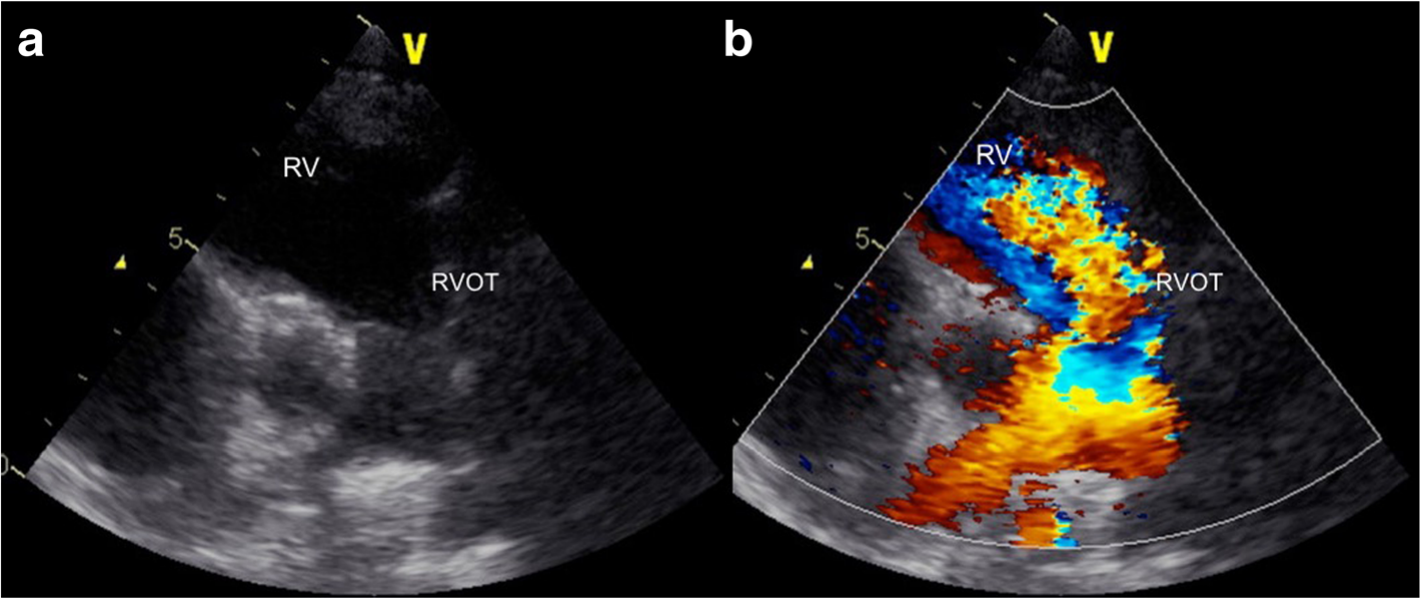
Echocardiogram in the short-axis view of aortic valve level showing severe pulmonary regurgitation. a: B-mode image; b: Color Doppler image. RV, right ventricle; RVOT, right ventricle outflow tract
